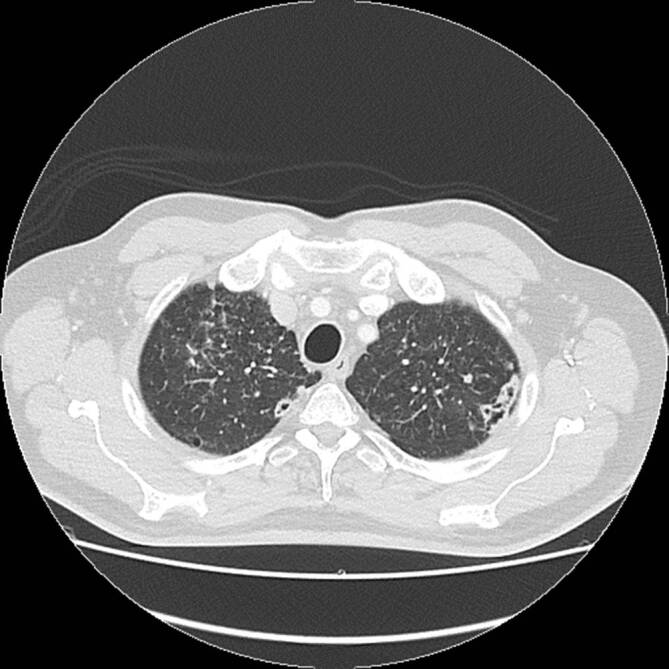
Aufgrund der Regredienz unter Antibiotikatherapie bei gleichzeitig nicht kontrollierter Rheumaaktivität wurde eine rheumatische Genese angezweifelt. Die Computertomographie (CT) des Thorax im April 2019 zeigte nunmehr einschmelzende Herde, zu deren pathogenetischer Einordnung vor Wiederaufnahme der Immunsuppression eine transbronchiale Kryobiopsie erfolgte. Diese ergab epitheloidzellige Granulome, die differenzialdiagnostisch nach direkter Mikroskopie sowie Hämatoxylin-Eosin-Färbung, Elastika-van-Gieson-Spezialfärbung, Grocott-Färbung, Ziehl-Neelsen-Färbung und Periodic-acid-Schiff-Reaktion als pulmonale Beteiligung der RA gewertet wurden. Die BAL zeigte ein mäßig granulozytäres und lymphozytäres Zellbild. Kulturell wurde kein Keim nachgewiesen. Ein interdisziplinäres Interstitial-lung-disease(ILD)-Board favorisierte nun nekrotisierende Rheumaknoten. Da keine infektiöse Ursache nachgewiesen werden konnte, wurde eine Intensivierung der Immunsuppression empfohlen.
